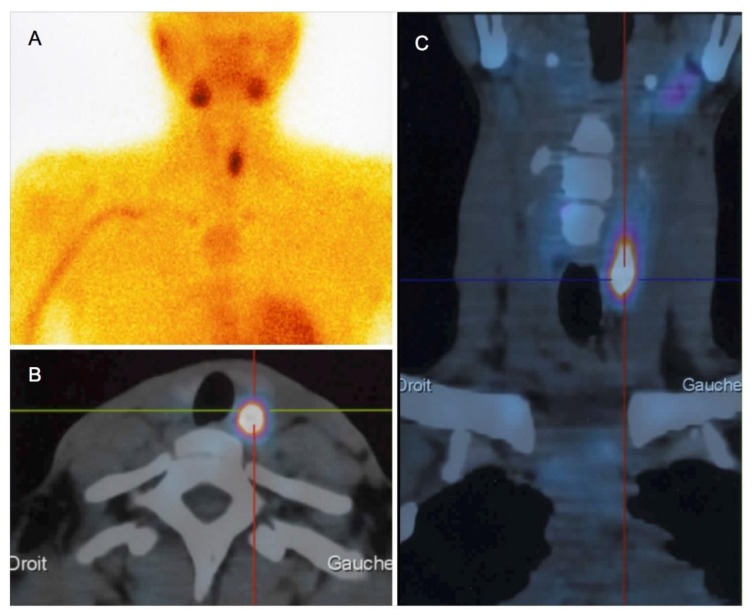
Tc99m-sestamibi scintigraphy (A) polled to computed tomography scans (axial (B) and coronal sections (C)) in a 30-year-old woman with pHPT and a 26-mm adenoma.
